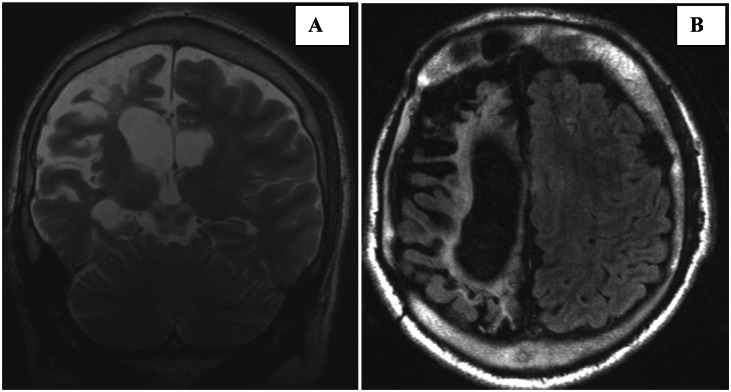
(A) Coronal T2-weighted MRI demonstrates right cerebral hemiatrophy with ipsilateral sulcal prominence and ex-vacuo dilatation of the lateral ventricle. (B) Axial FLAIR image shows dilatation of the right lateral ventricle with periventricular T2/FLAIR hyperintensity, consistent with gliotic changes.
